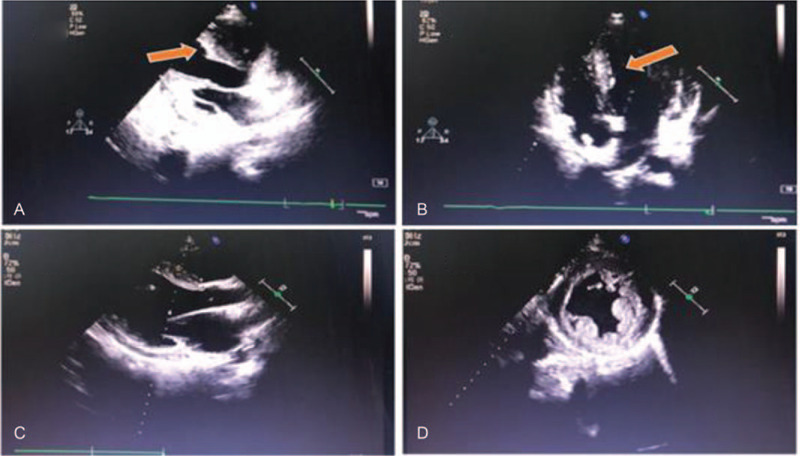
Echocardiography images. A, B: Thickening of the interventricular septum in the (A) long axis section of left ventricle and (2b) 4 chamber section. Initial septal thickness was 17 mm and initial thickness of the left ventricular wall was 13 mm. C, D: Echocardiogram showing that myocardial densification was not complete, that the left atrium and left ventricle were enlarged, the left ventricular beat was diffusely weakened, and systolic function was reduced. The left ventricular diastolic diameter was 68 mm, the left ventricular wall thickness was 9 mm, the septal thickness was 12 mm, and EF was 46%.
